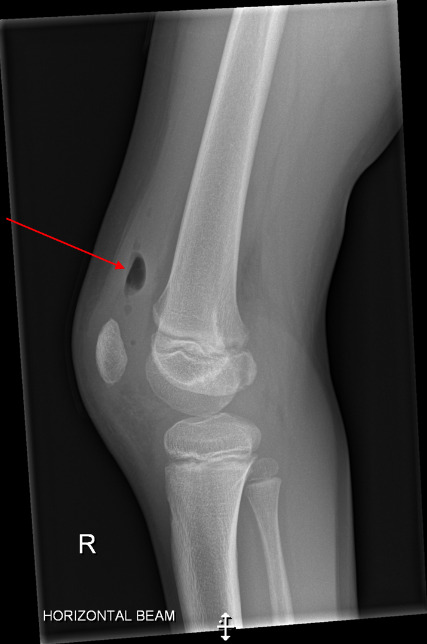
Lateral X-ray of the patient’s right knee showing gas in the suprapatellar pouch on day 2 (case 1).
